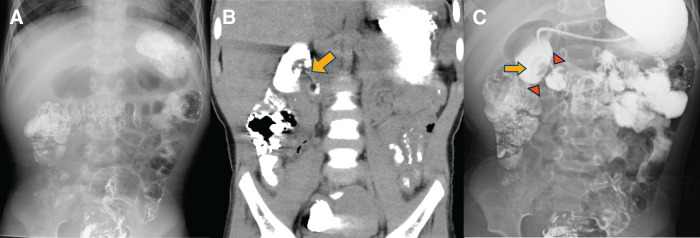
(A) Abdominal X-ray at admission to our institution showed an abnormal gastric bubble and duodenal bulb dilation, suggestive of upper gastrointestinal tract stenosis. (B) Abdominal CT image showing a mushroom-shaped foreign body in the descending portion of the duodenum (arrow). (C) An upper gastrointestinal contrast image showing a hemispherical foreign body in the descending portion of the duodenum (arrow) and a contrast filling defect suggesting the stenosis (arrowheads) at the same site. Contrast medium passed smoothly beyond the descending portion of the duodenum, with no evidence of obstruction in the distal segment.
